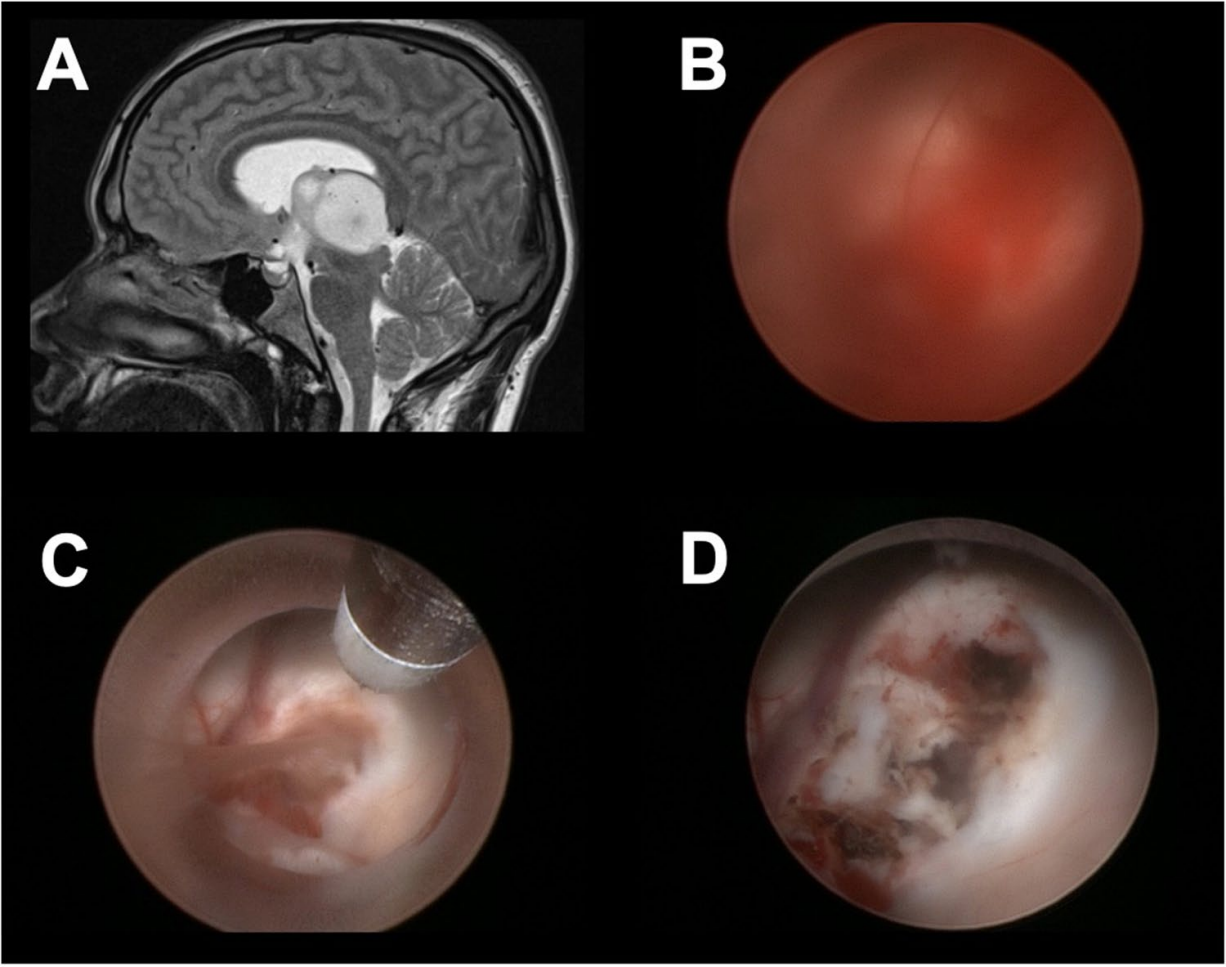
Example of the application of the small chamber technique. Preoperative MRI of a 18-year patient with a glioblastoma (A). A grade IV hemorrhage occurred during the tumor biopsy and lasted for 11 min. Irrigation did not improve the vision and no identification of the bleeding source was possible (B). The small chamber technique was applied to obtain a clear vision and identification of the injured vessel. The bipolar electrode was used for coagulation (C). The hemorrhage stopped and the procedure was finished including an additional ETV (D)
